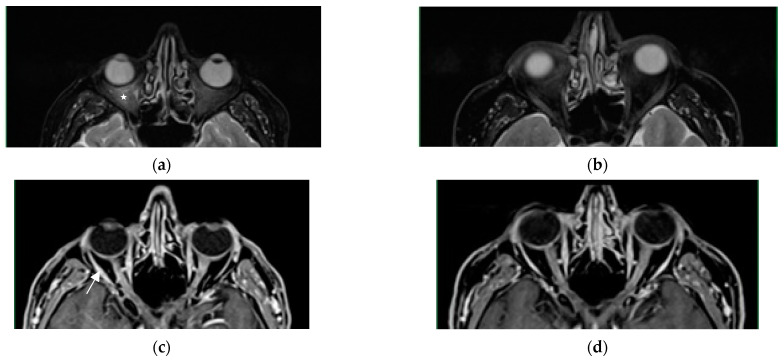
T2-weighted images with fat saturation, axial plane—(a,b). Moderate edema of the intraconal fat secondary to optic neuritis ((a)—white asterisk). Resolution of edema on follow—up examination (b). T1-weighted image with gadolinium contrast administration, axial plane—(c,d). Heterogeneous optic nerve enhancement, more significant on the right side ((c)—white arrow). Marked partial regression of lesions on follow-up examination (d).
